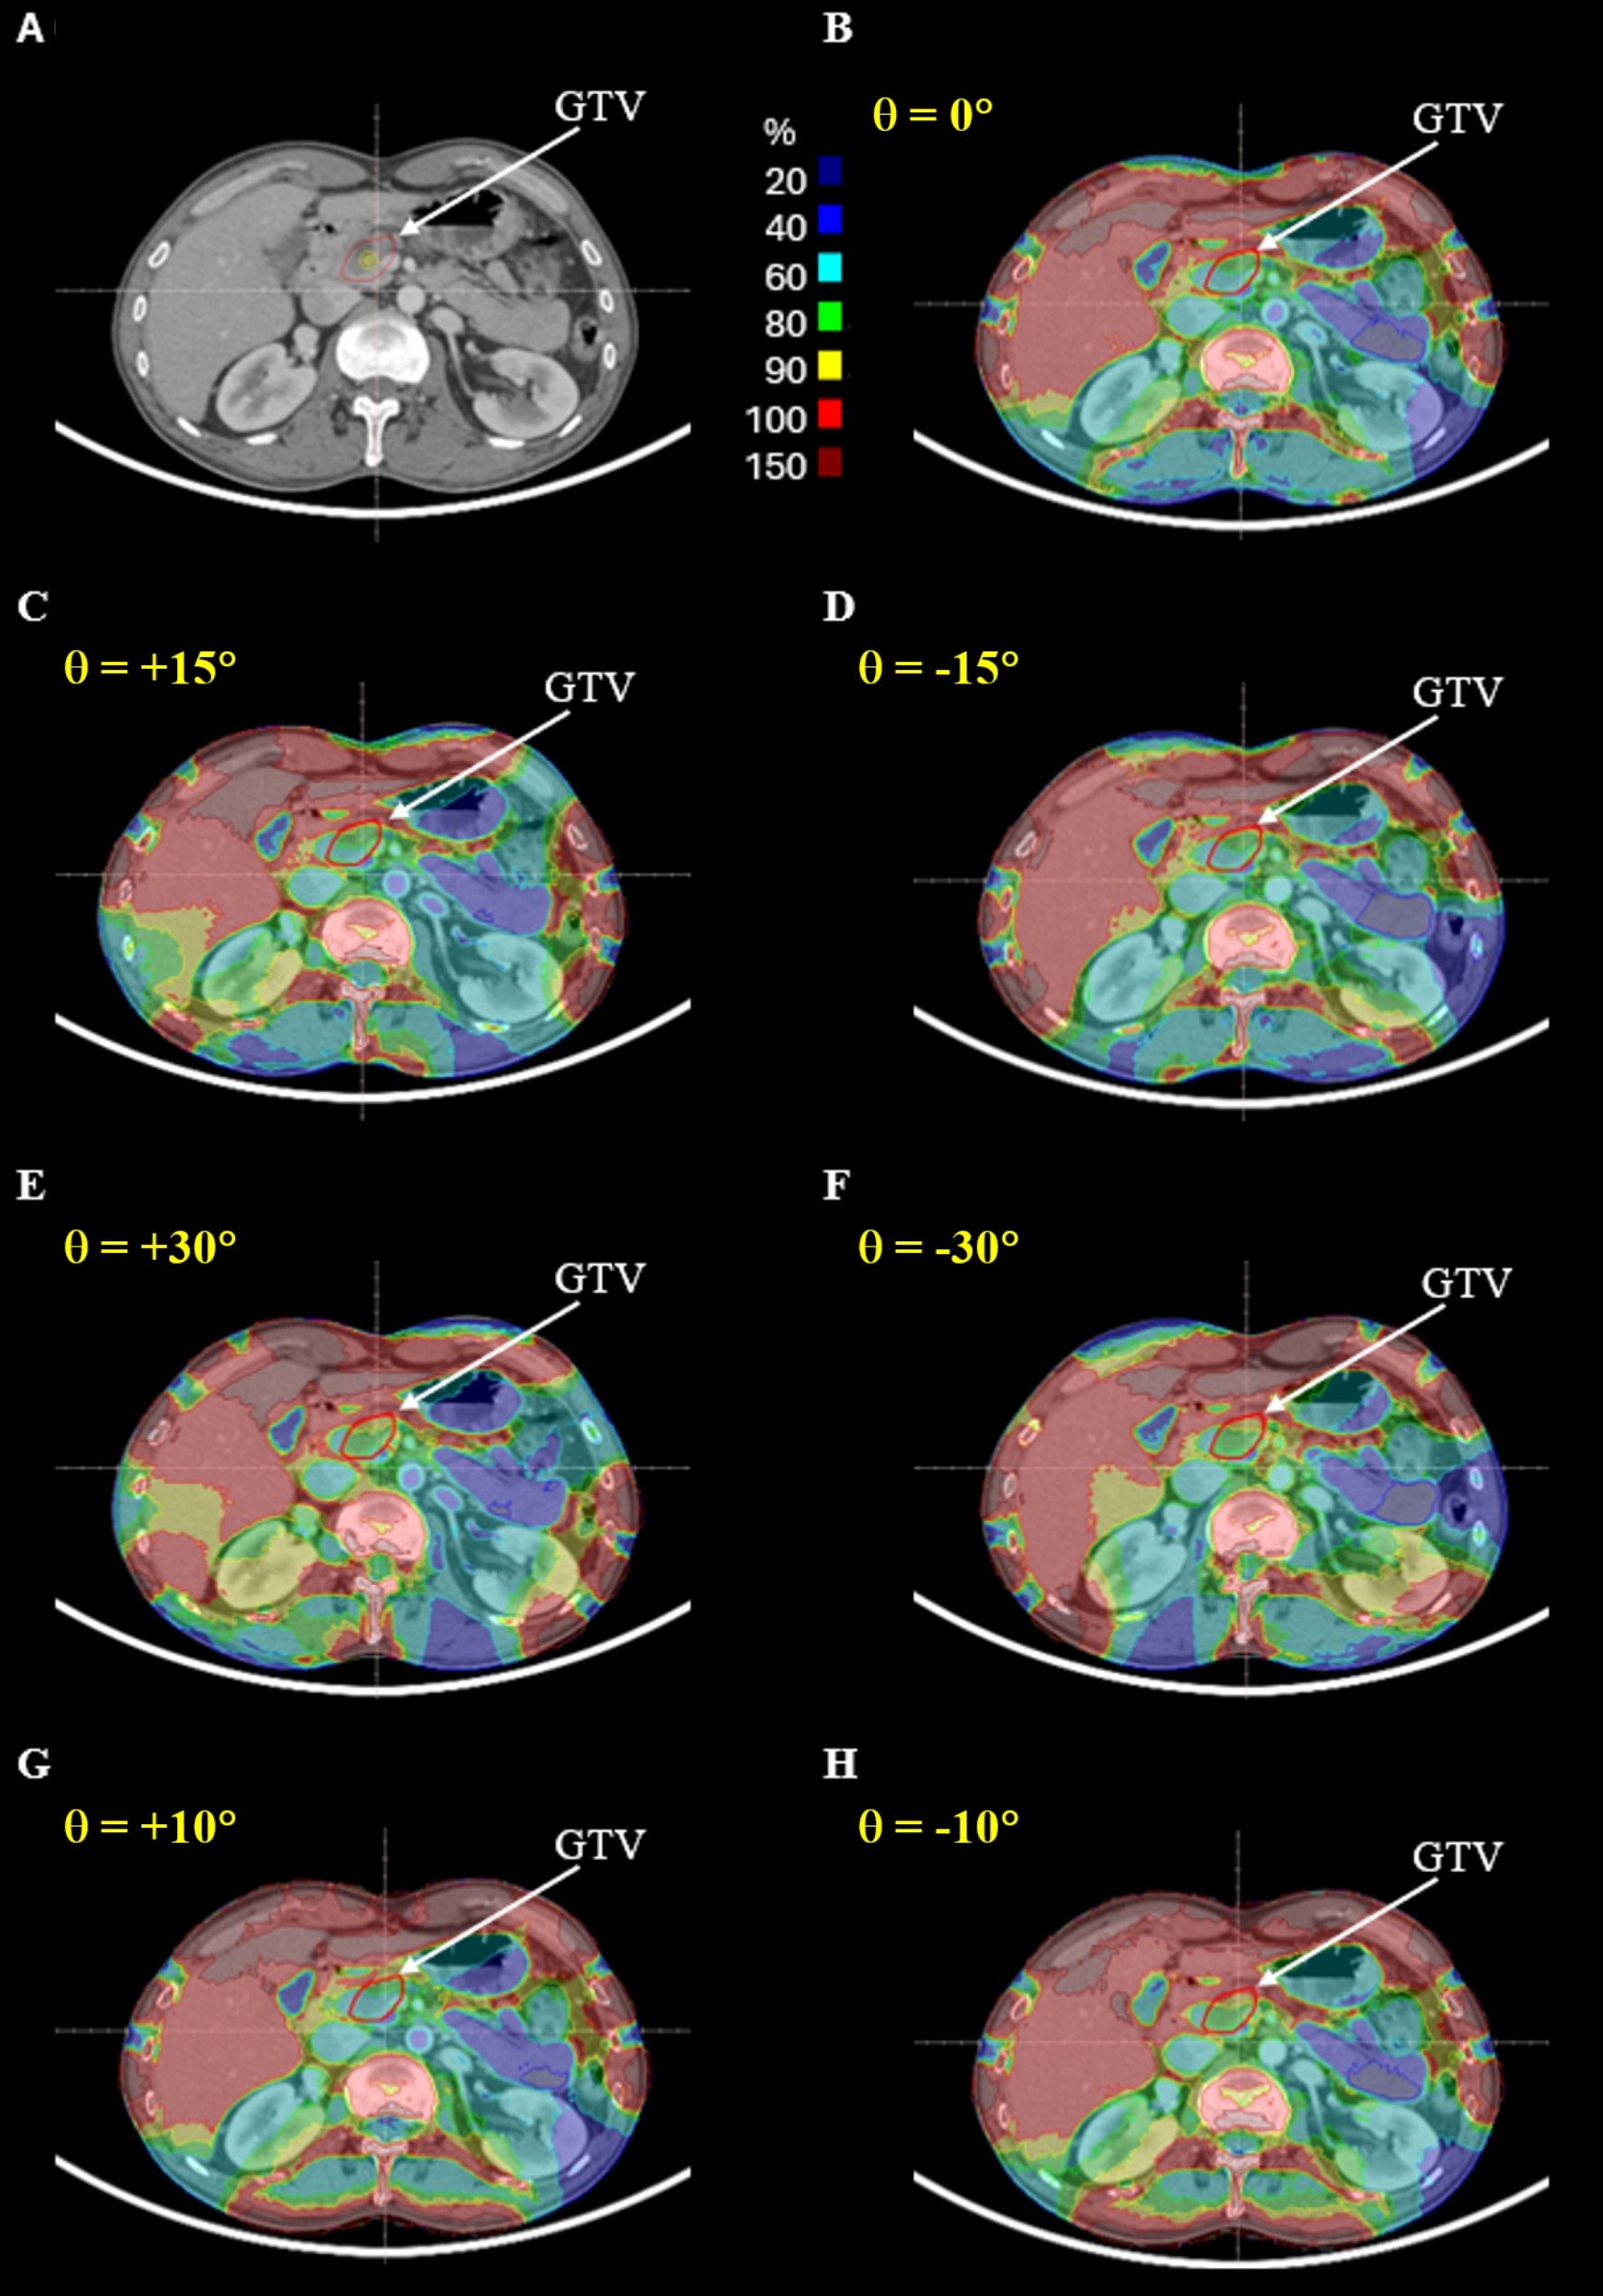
Dependence of electric field distribution on electrode locations for representative patient 1. A Axial CT image at the center of the tumor. B Reference plan. C Trial plan rotated θ = +15⁰ in the axial plane. D Trial plan rotated θ = -15⁰ in the axial plane. E Trial plan rotated θ = +30⁰ in the axial plane. F Trial plan rotated θ = -30⁰ in the axial plane. G Trial plan rotated θ = +10⁰ in the sagittal plane. H Trial plan rotated θ = -10⁰ in the sagittal plane.
